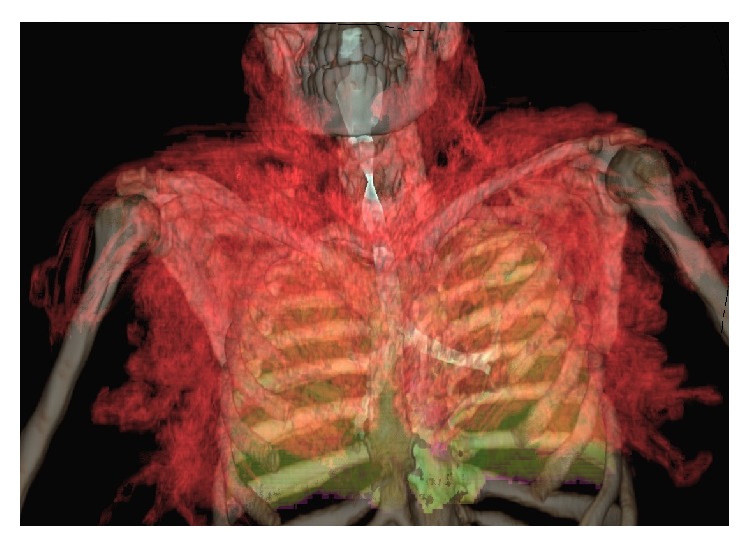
3D reconstruction from images of the cervical-thoracic CT obtained emergency shows the distribution of emphysema in relation to other structures aeric content. Emphysema is shown in red; the trachea and bronchi are shown in blue, light green lung.
